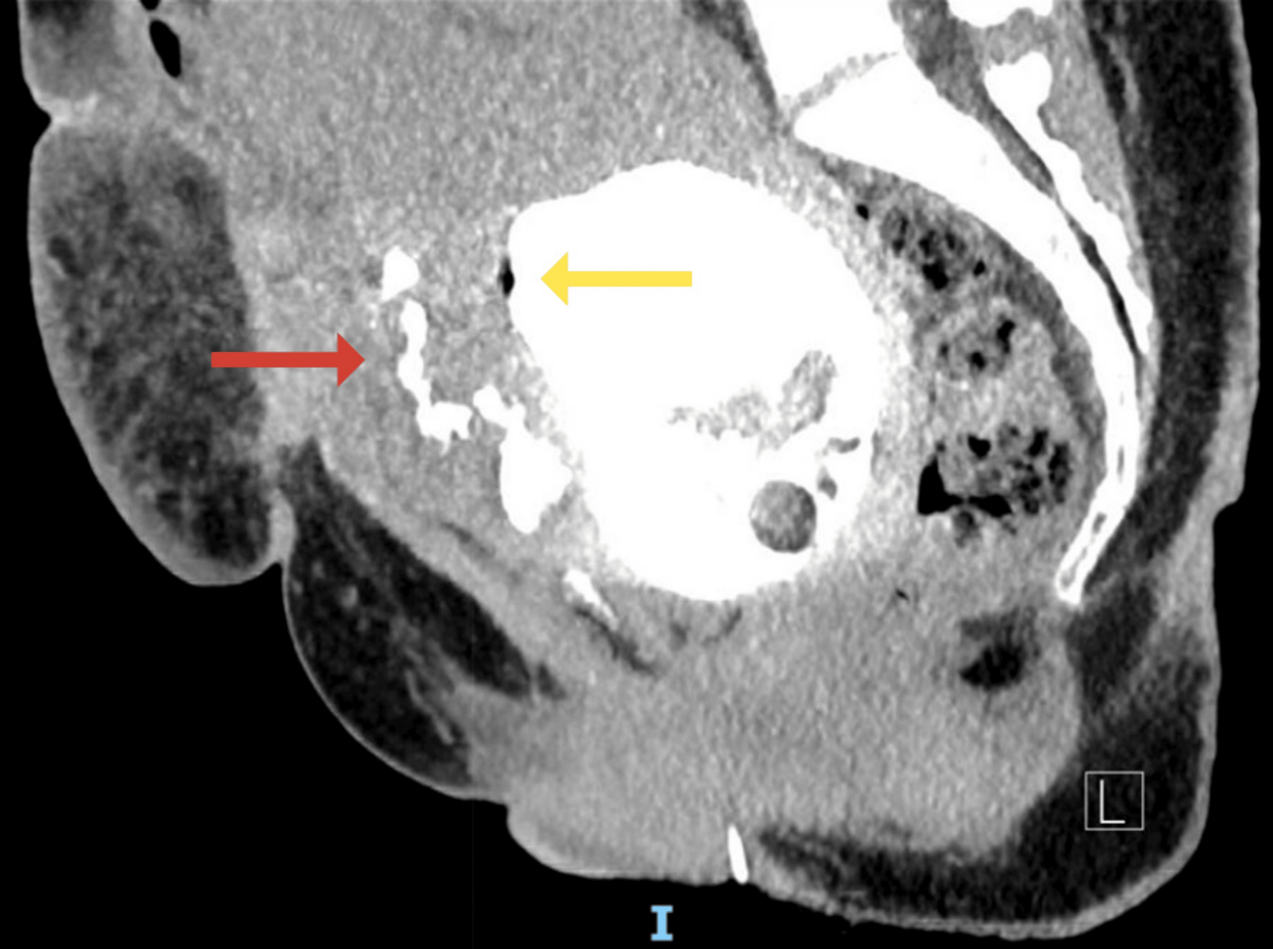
Sagittal view of the Initial CT cystogram demonstrating a small partial tear in the anterior wall of the urinary bladder measuring approximately 4 mm in diameter (yellow arrow) associated with extravasation of contrast. Redemonstration of an organized collection seen anterior to the bladder and lower uterine segment beneath the anterior abdominal wall with contrast leaking through this collection (red arrow).
